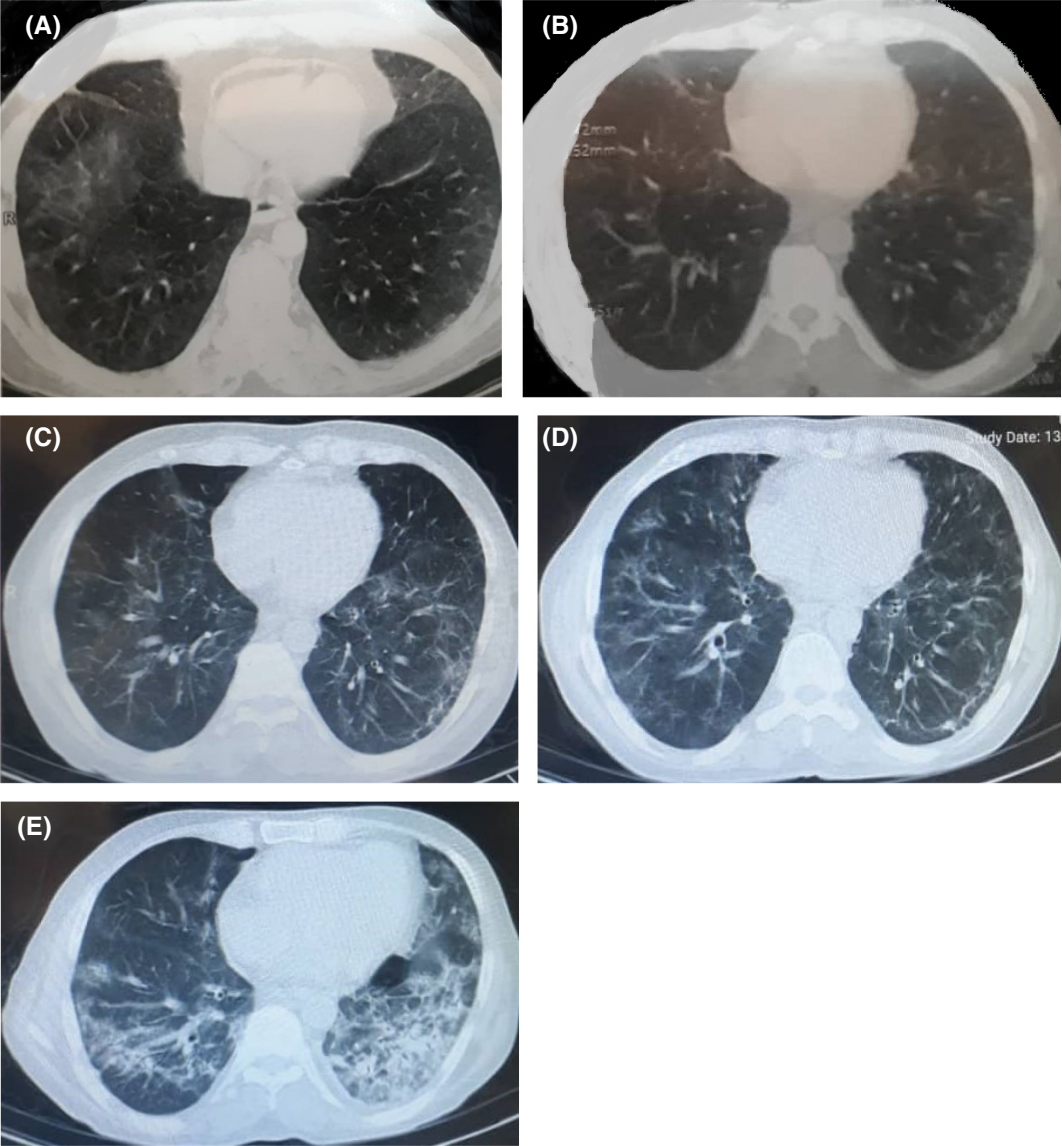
Chronological changes in CT scan
